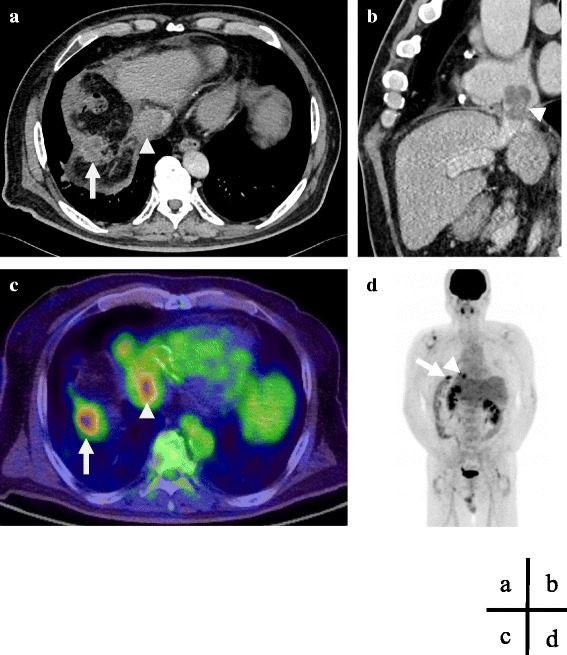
Preoperative imaging studies. a Abdominal enhanced computed tomography revealed the tumor in the diaphragm (*arrows*) and the tumor thrombus (*arrowhead*). b The tumor thrombus extended from the retrohepatic inferior vena cava to the right atrium. c Positron emission tomography revealed the physiologic uptake of 18-fluorodeoxyglucose in both the diaphragm and right atrial tumor, d suggesting no remote metastasis
